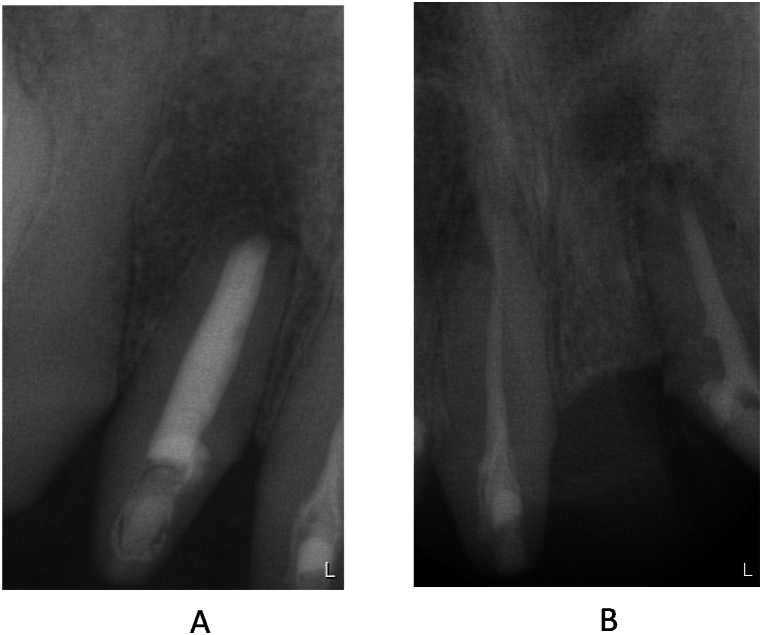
Periapical X-ray about the 12 tooth (A) and about the 11 and 22 teeth (B) after 31 month of the surgical procedure.
